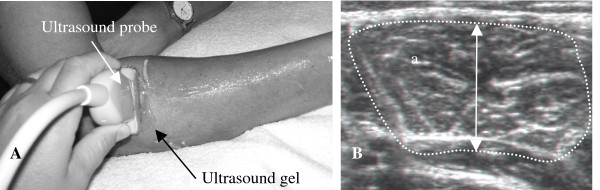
US measuring point. (A) Position of the probe for ultrasound measurements of the EDC, 15% distal of the EDC origin. (B) Transverse US image obtained at the measurement position (contracted muscle). The circumference is shown by the dotted line, the cross-sectional area is the area within the line, and the muscle thickness is indicated by the double-headed arrow.
